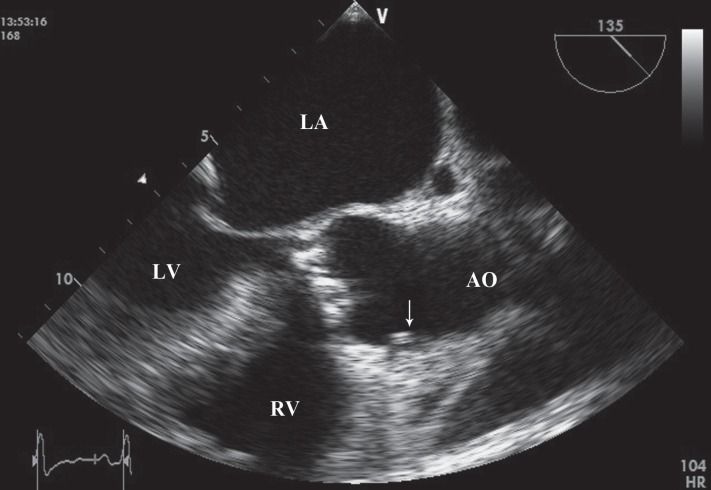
Vegetation on the anterior aortic wall prolapsing to the proximity of the right coronary ostium on transesophageal echocardiography (arrow)
